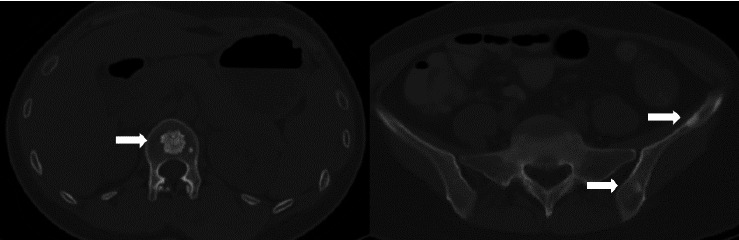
Current CT image of an osteoma in the left ilium and thoracic vertebral bone.
